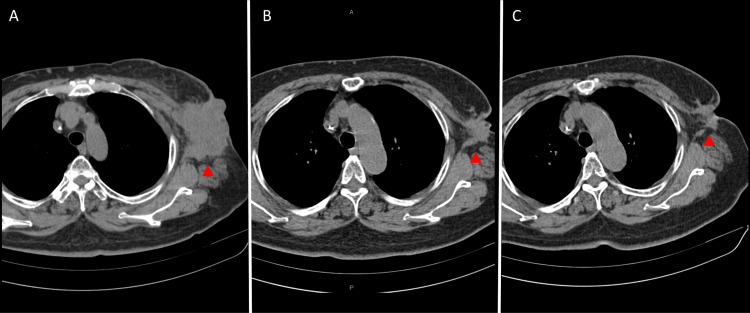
Time series of CT findings of the chest, showing the axillary tumor (▲) and its reduction in size over time following the initiation of immunotherapy-based combination therapy (A) At the first visit, when immunotherapy-based combination therapy was initiated. (B) Six months after the start of treatment, showing partial response. (C) One year later, with continued monitoring and ongoing treatment.
